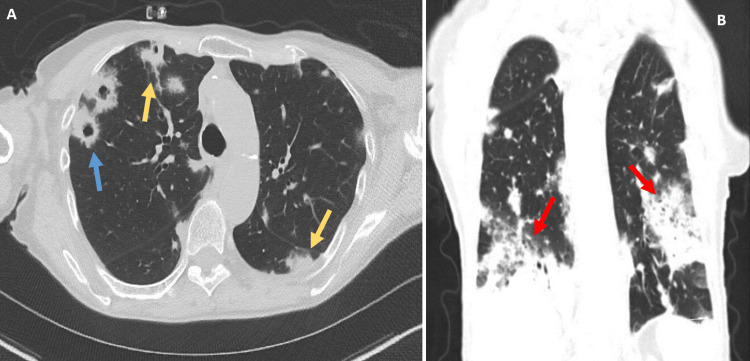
CT of the Chest Findings A: varying-sized nodules, some of which are cavitary (blue arrow) with ground glass opacities (yellow arrows). B: coronal view of more consolidated lesions and nodules (red arrows) with bilateral distributions. CT: computed tomography
